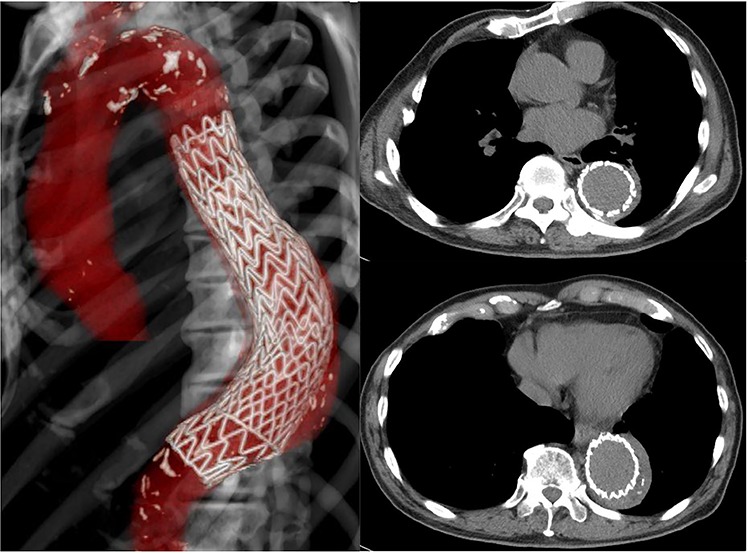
CT 15 months later shows regression of the aneurysm to 53 mm in diameter.
